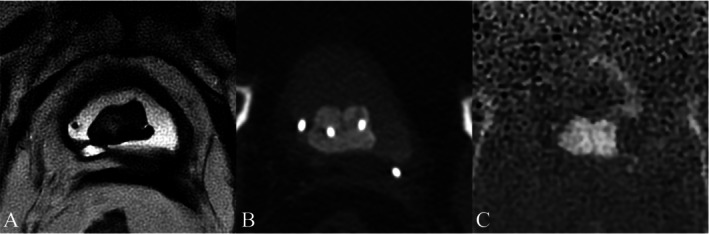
Axial T2‐weighted MRI (A) demonstrates a hypointense lesion within the urinary bladder at the distal tip of a DJ stent in a patient previously treated for cervical squamous cell carcinoma. Corresponding axial CT in bone window (B) and zero echo time (ZTE) MRI (C) confirm dense calcification within the lesion, consistent with a bladder calculus.
